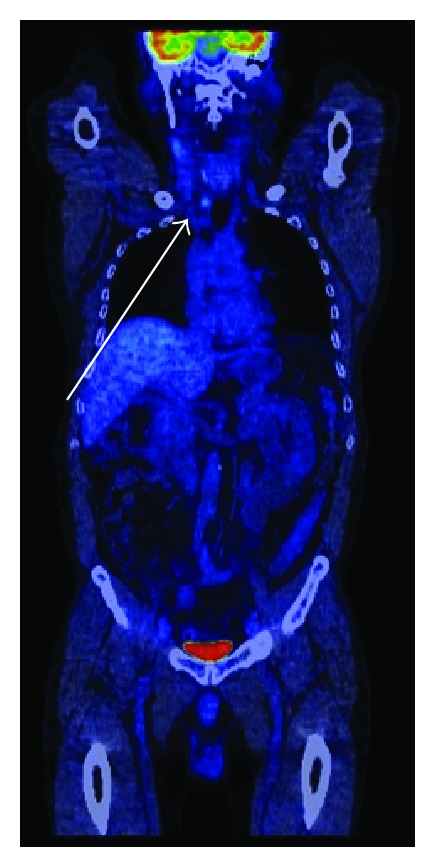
F18-FDG-PET-CT after the therapy in June 2013, showing the persistency of the tumor in the upper mediastinum with high glucose uptake and SUV_lbm_ 3.67.
